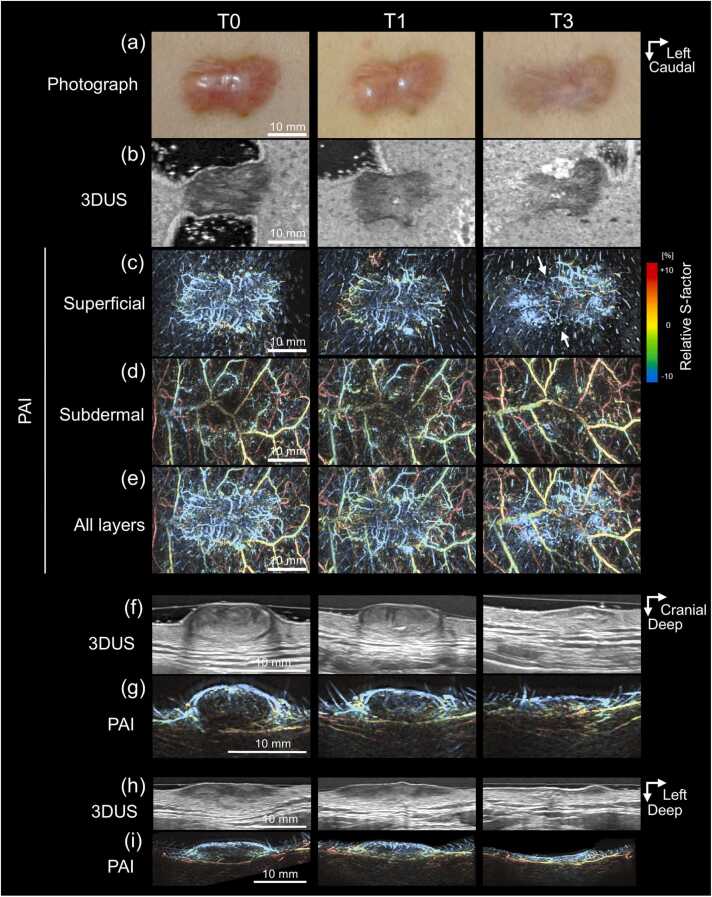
Longitudinal changes in Case 1. Before treatment, the keloid appeared elevated and erythematous and became flattened after treatment (a). Ultrasound images (b, f, h) visualize the keloid as a hypoechoic region, exhibiting an elliptical profile in the sagittal view (f) and a lenticular profile in the axial view (h). After treatment, the hypoechoic area decreases in size, and its boundary with the surrounding dermis becomes indistinct in the horizontal view (b). Photoacoustic images with color-coded relative S-factor values (c–e, g, i) reveal radial clusters of microvessels with uniformly low oxygen saturation surrounding the keloid surface. After treatment, the number of central microvessels decreases (arrows). The subdermal layer (d) contains a vascular network with heterogeneous oxygen saturation, and both the spatial distribution and oxygenation levels show minimal change after treatment.
